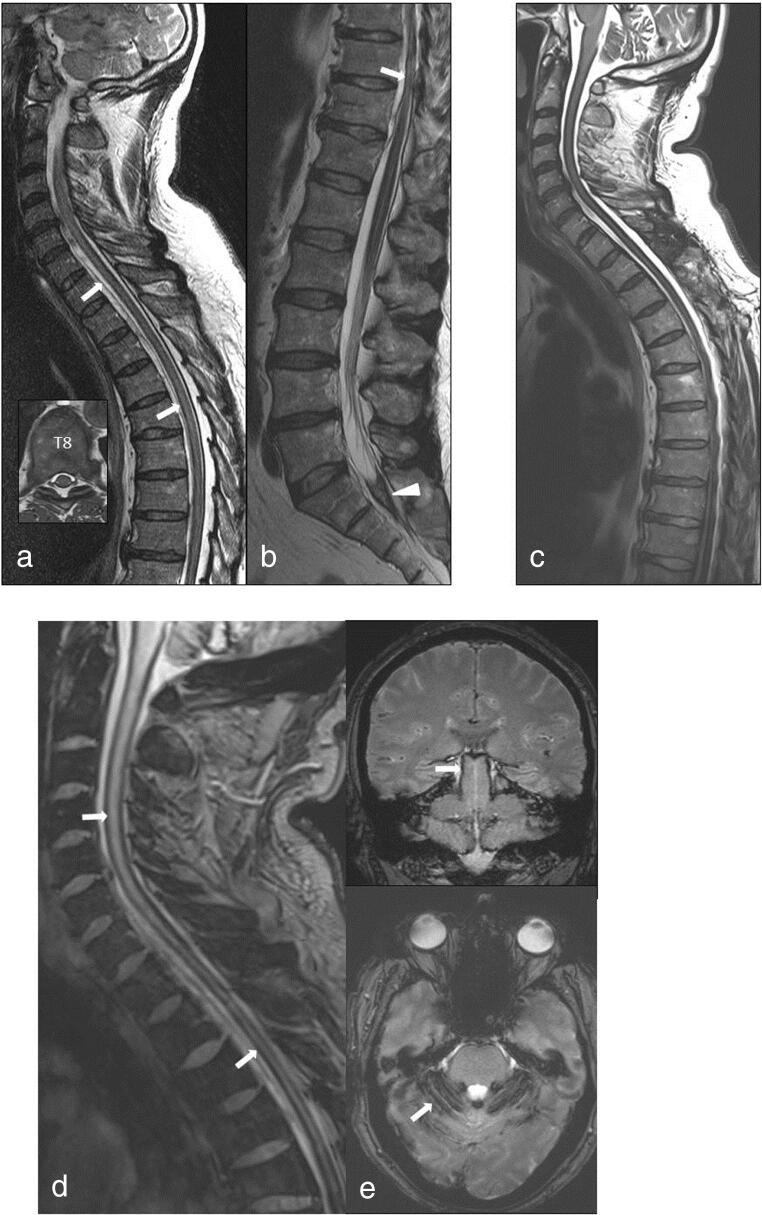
Preoperative sagittal and axial (inset) T2-weighted MR images of the cervicothoracic and lumbar regions (a, b) reveal extensive anterior intraspinal fluid collection from C5/6 to T 11 (arrows). Note hypointense formation of the distal thecal sac due to hemosiderin deposits at S1/2 (arrowhead). Postoperative sagittal T2-weighted MR images (c) 13 months after repair of the T1/2 dural tear shows persisting resolution of the fluid collection. Gradient echo MR images (d, e) clearly detect hemosiderin depositions along and around the cord associated with cord atrophy, as well as along the cerebellar folia, around midbrain and pons (arrows)
